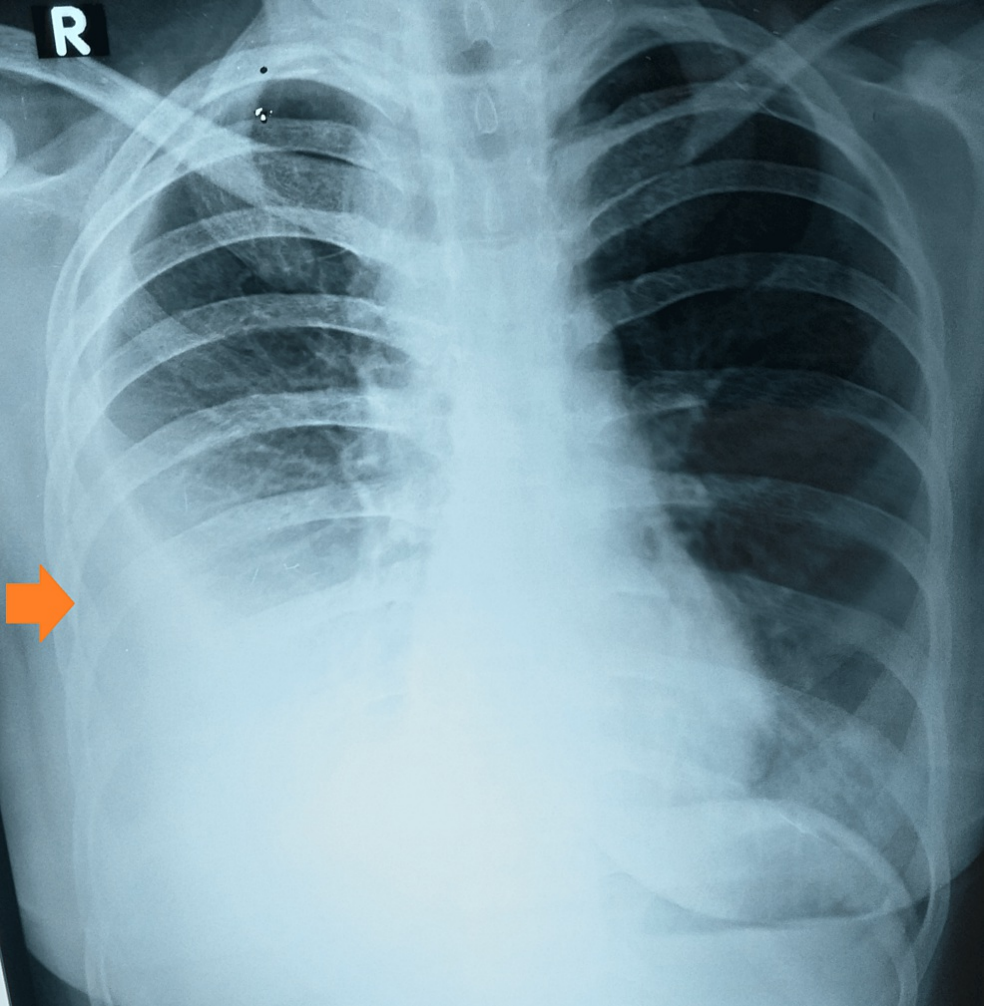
Chest radiograph (P-A) view suggestive of right-sided pleural effusion P-A: postero-anterior
